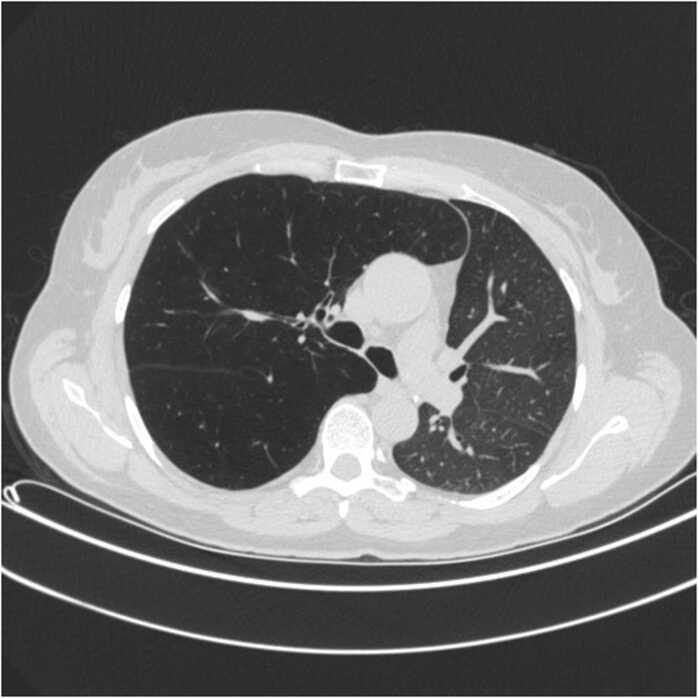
Preoperative chest CT depicting the transplanted left lung and the hyperinflated right native lung compressing the mediastinum toward the left. CT, computer tomography.
